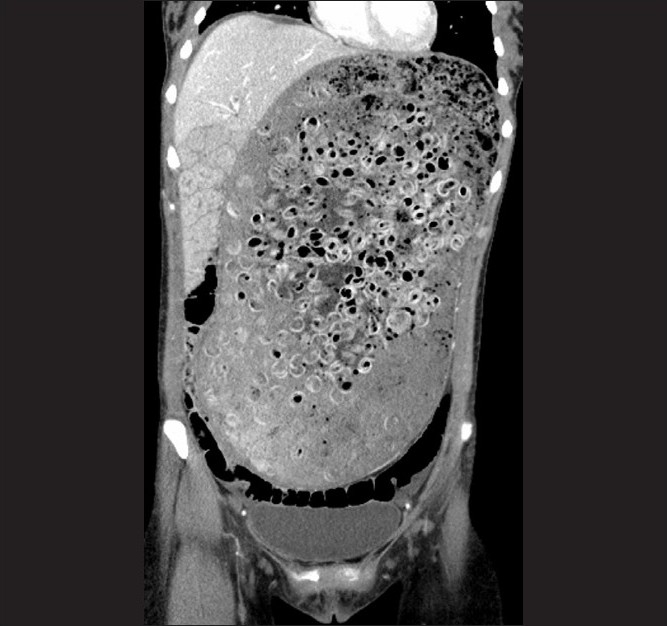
Coronal image of an abdominal CT scan, which reveal a distended stomach containing air and circular radiodense material, which was later revealed to be pasta
